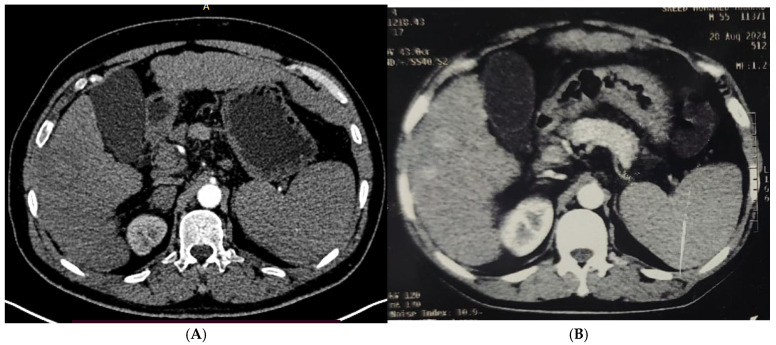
(A). Triphasic CT abdomen (arterial phase) axial cuts revealing, in right lobe segment V, two adjacent focal lesions with homogeneous enhancement (before MWA). (B). The same lesion (arterial phase) axial cuts one month after MWA.
